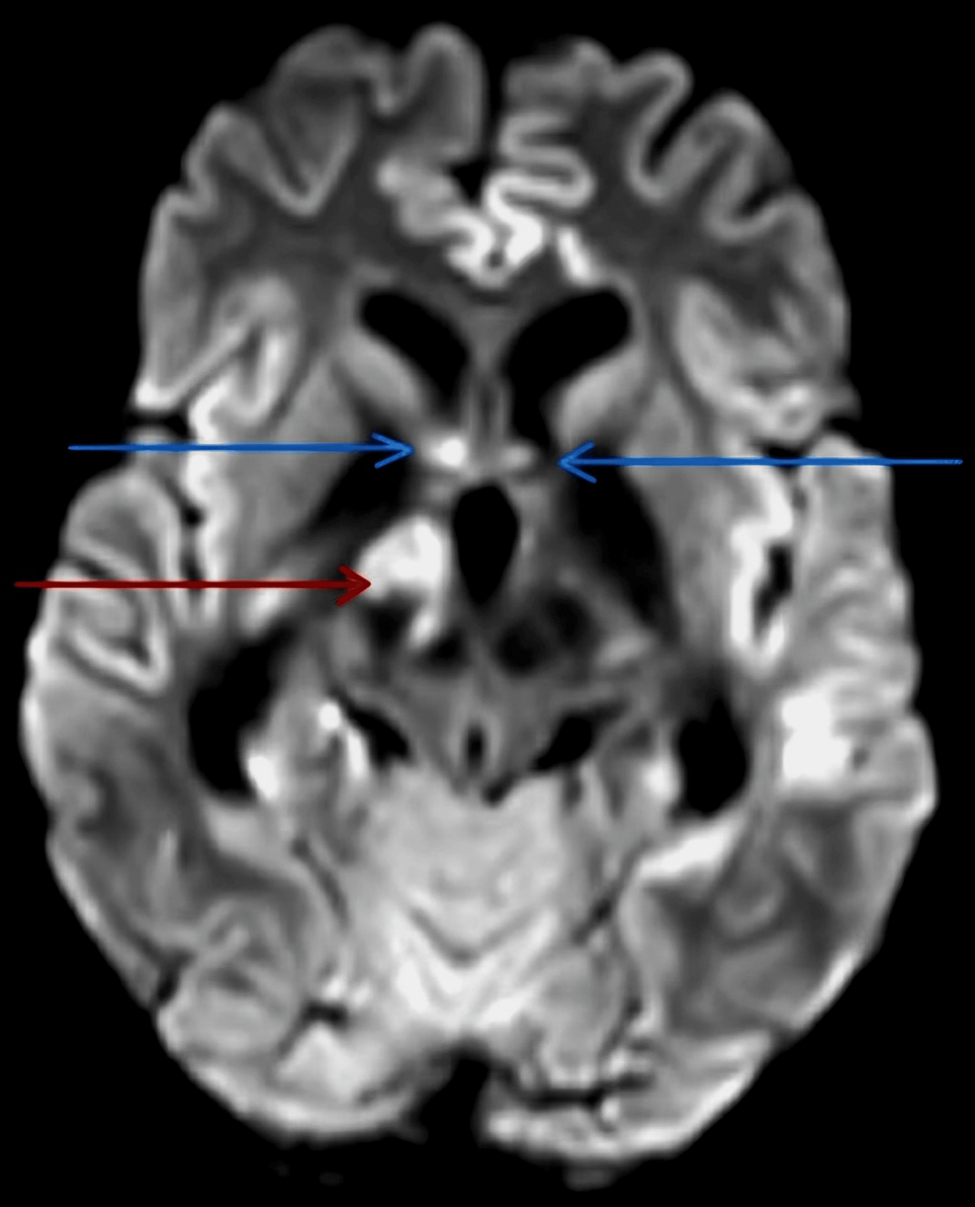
MRI brain showing areas of restricted diffusion in the right thalamus (red arrow) and bilateral basi-frontal region, adjacent to bilateral temporal horns of lateral ventricles (blue arrows). MRI- Magnetic Resonance Imaging
